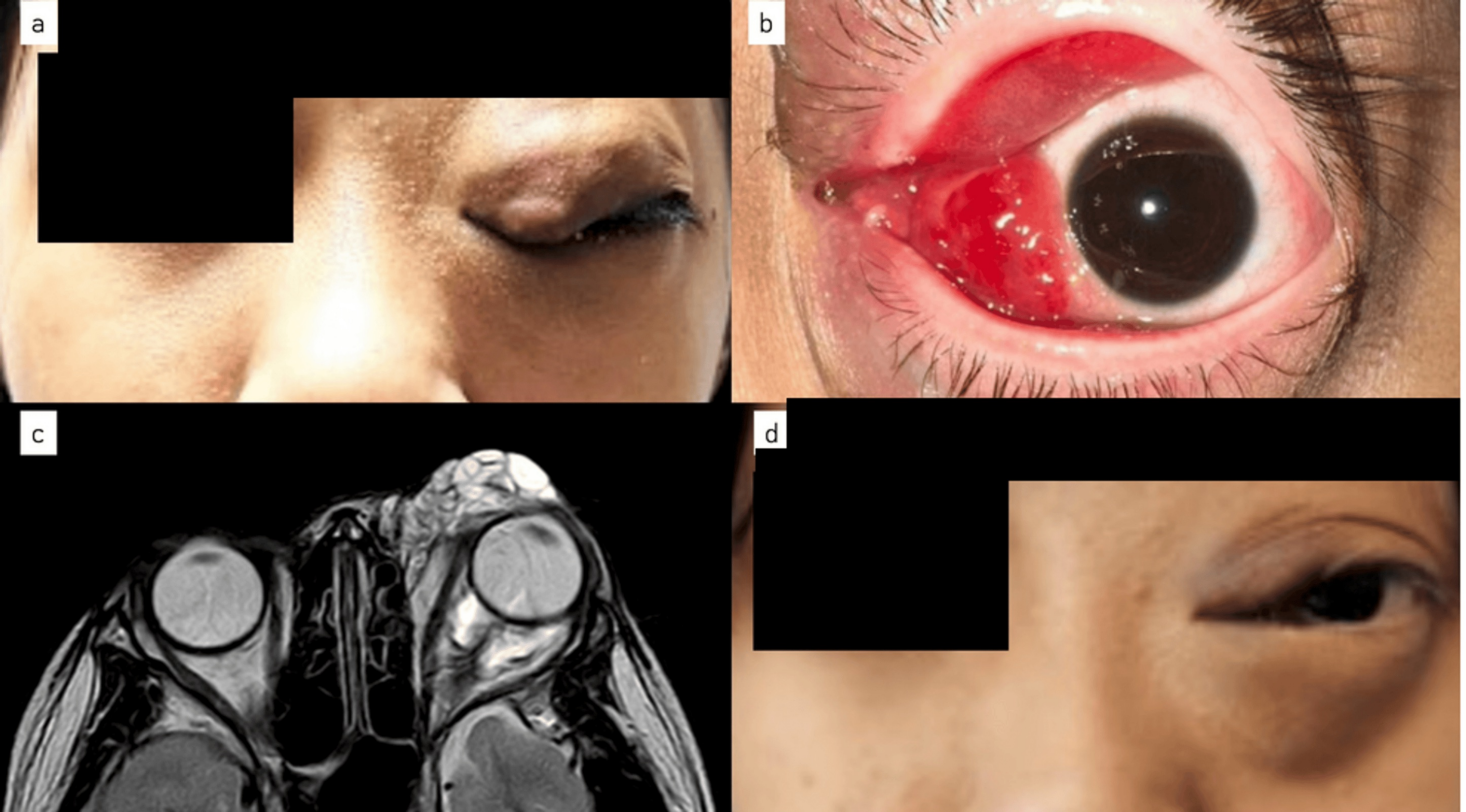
Case 1 on presentation showing left eye proptosis (a) with lesions involving the upper and lower lid (b). MRI orbit showing multiloculated lesions extending into the medial extraconal and retro-orbital space (c). Six weeks post the third intralesional bleomycin showed a good response to treatment with a noticeable decrease in lid swelling (d).
